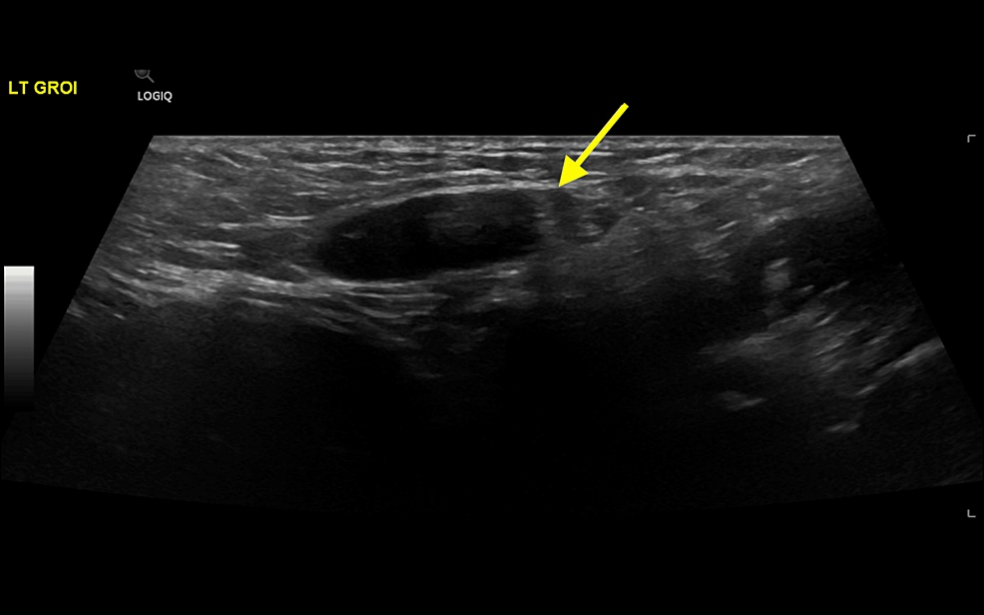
Ultrasound image of the left groin shows a small inguinal testicle (yellow arrow).
